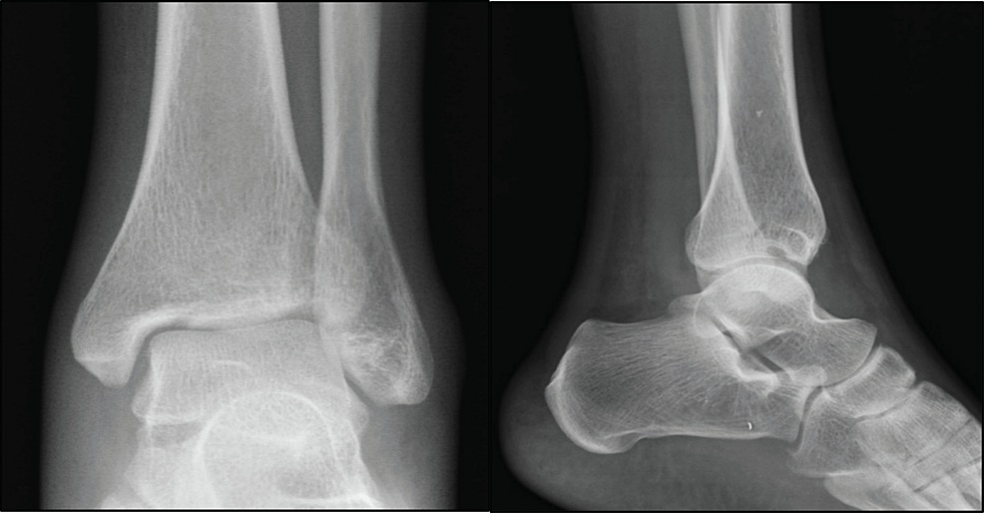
X-ray at presentation showing minimally displaced tibial plafond fracture
